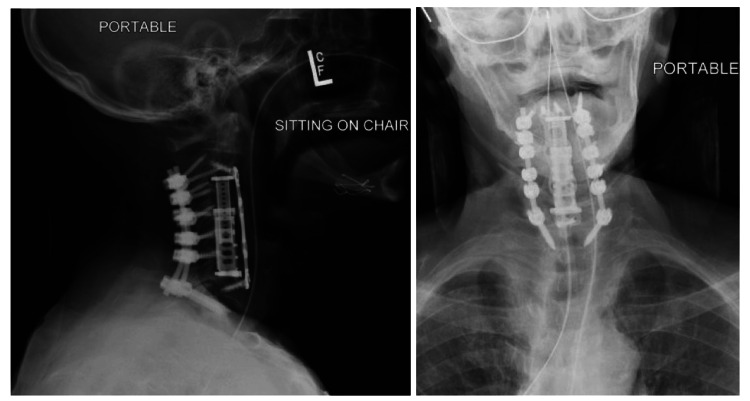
Postoperative X-rays indicating correct hardware placement
